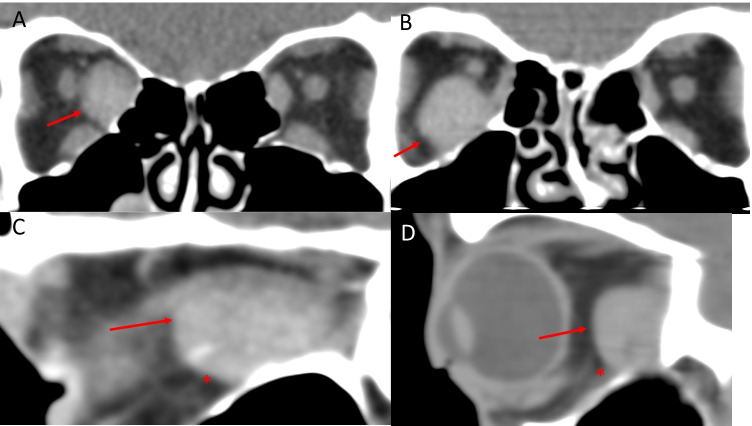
Two cases of Spontaneous haemorrhage related to extraocular muscles. Case 1—coronal (A) and sagittal (C) CT scans showing a large, well-defined mass in the right medial rectus with an anterior rounded border (arrow) and posterior tapering edge (*) towards the orbital apex. The sagittal image has a ‘bleached whale’ appearance suggestive of intramuscular haemorrhage. Case 2—coronal (B) and sagittal (D) CT scans showing a large, well-defined mass in the right inferior rectus with an anterior rounded border (arrow) and posterior tapering edge (*) towards the orbital apex
